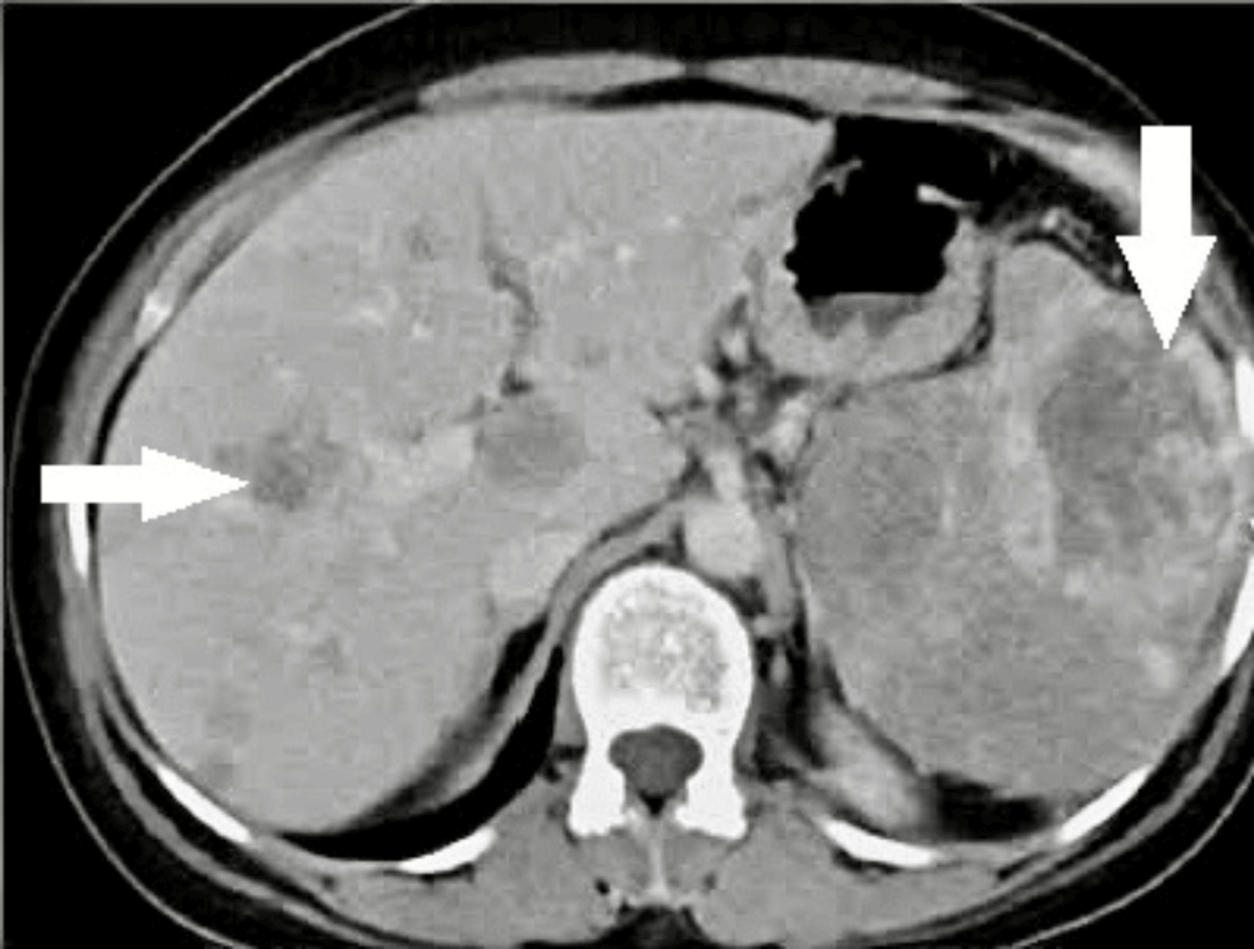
Primary splenic angiosarcoma with spontaneous rupture Contrast-enhanced CT scan showing multiple heterogeneous lesions in both liver and spleen with hemoperitoneum. The white arrows show the liver metastases and splenic lesion.
